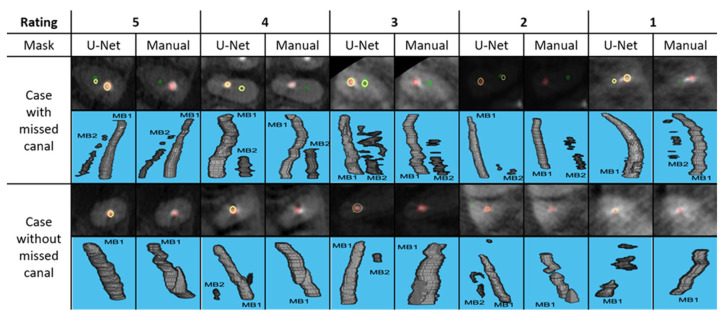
Typical examples of each segmentation rating containing CBCT axial cross section with segmentation overlaid (top) and 3D view (bottom). MB1 is obturated canal, depicted in red and MB2 is unobturated canal, depicted in green. The yellow ring represents the location of manual segmentation relative to U-Net generated segmentation.
